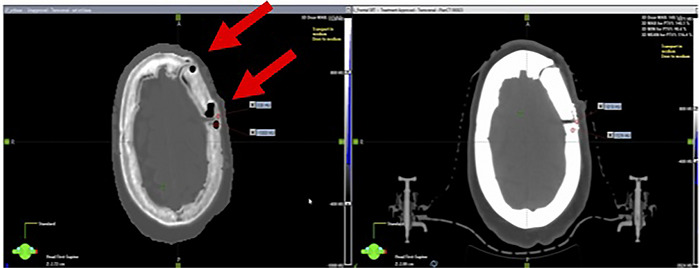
Comparison between sCT and CT images where the anatomy of the patient was altered and surgical meshes were present.
